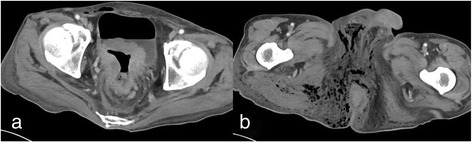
Computed tomography scan showing a rectal tumor invading the bladder (a) and abscess formation with emphysema in the pelvis and perineum (b)
